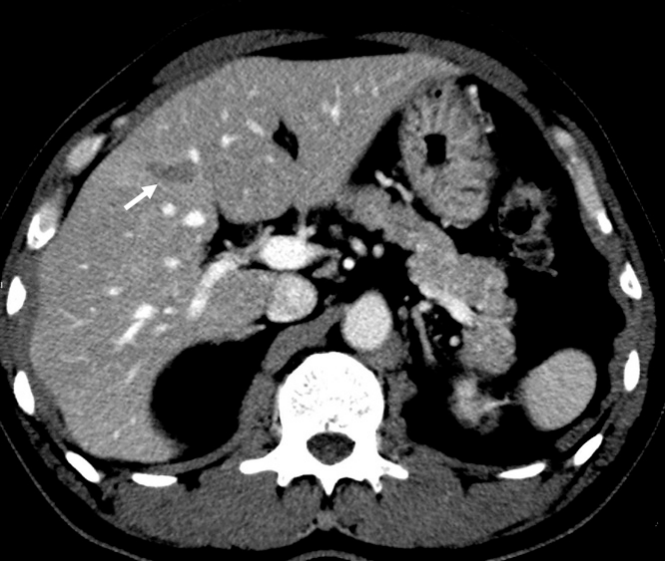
The CT image obtained 10 months later shows that the nodule migrates slowly upward and anteriorly in the liver (arrows).
